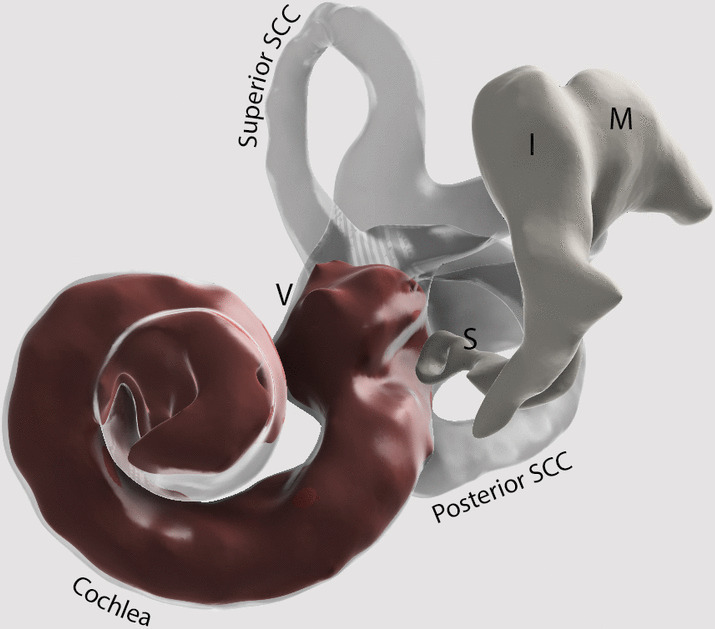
The intralabyrinthine schwannoma (in red) in the left inner ear, seen in close relation to the ossicular chain. SCC: semicircular canal; V: vestibule; S: stapes; I: incus; M: malleus. Image created from fused CT and T2-weighted MRI images, using 3D Slicer (open source) and Fusion 360 (Autodesk, San Francisco, CA, USA) [11]
